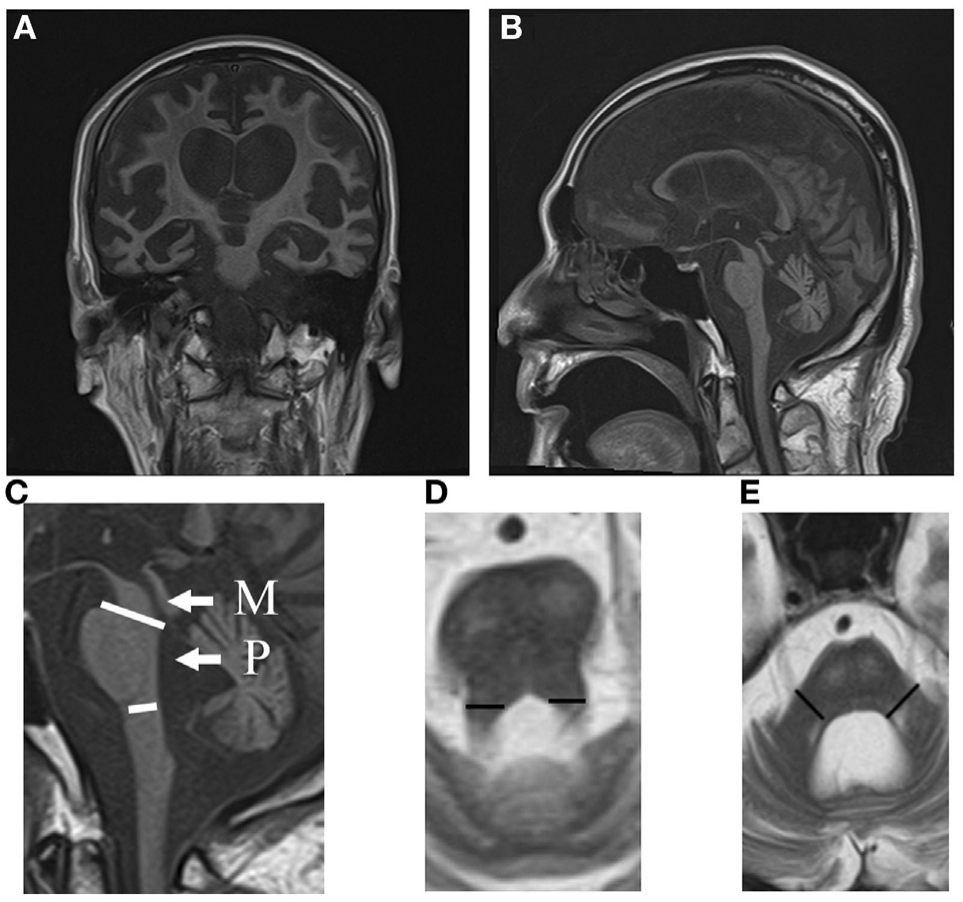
MRI coronal (A) and sagittal (B) T1 images (TR 540 ms, TE 15 ms) of the MSA patient in August 2016. Note the atrophy in the caudal pons. The midbrain and cerebellum are atrophic. (C) Areas of the midbrain (M) and pons (P) are shown. (D) The width of the superior cerebellar peduncles (segments) is shown. (E) The width of the middle cerebellar peduncles (segments) is shown. MSA, multiple system atrophy; MRI, magnetic resonance imaging.
